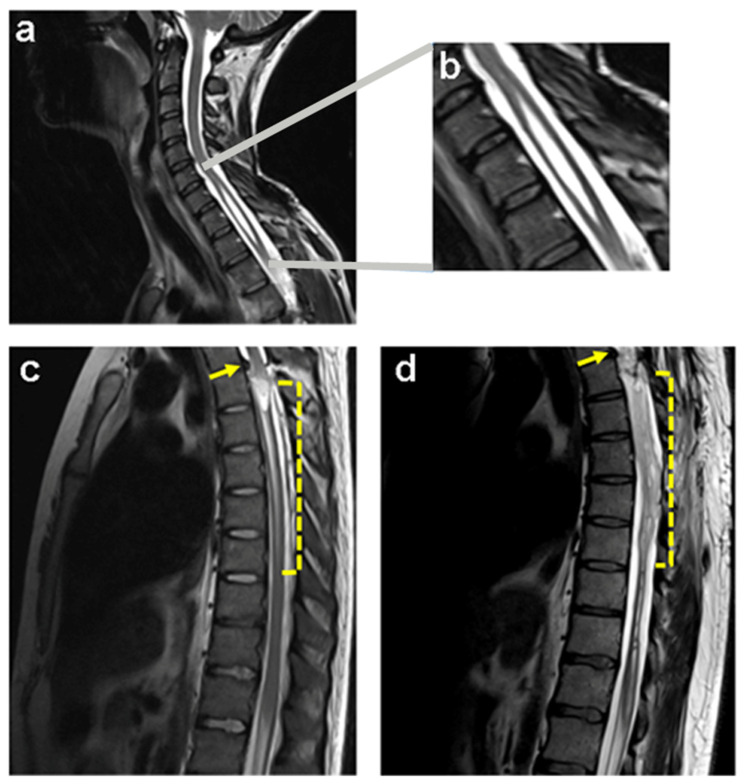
Subacute and chronic lesions of the spinal cord in human patients observed by magnetic resonance imaging. Syringomyelia cyst in a child due to idiopathic causes (a) shows the enlargement of the cavity within the spinal cord (b). The dotted areas (c,d) indicate a decade of evolution in a patient from subacute injury (c) to chronic spinal injury (d) produced by traumatic disk herniation at the second to third vertebral thoracic levels, T2–T3 (see arrows). Note the formation of syringomyelia below the lesion at the beginning (c) and how ten years later, the spinal cord becomes necrotic (d).
